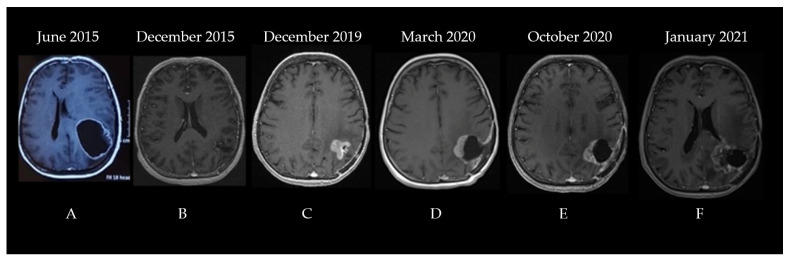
The patient’s disease progression showcased through serial brain MRI imaging ((A)—left frontoparietal process with a heterogeneous signal and structure, irregular margins, and both solid and cystic components surrounded by moderate edema, which compressed and tractioned the trigon and body of the left lateral ventricle upward, (B)—left parietal postoperative cavity with discrete wall contrast enhancement, minimal traction over the occipital horn of the left lateral ventricle, and midline structures in normal position, (C)—vascularized tumoral tissue on the medial and superior wall of the postsurgical resection cavity with perilesional edema, (D)—postoperative cavity and residual tumor after second surgery, (E)—postoperative cavity after second surgery with progression of residual tumor, (F)—further progression of residual tumor coupled with radiation induced changes).
